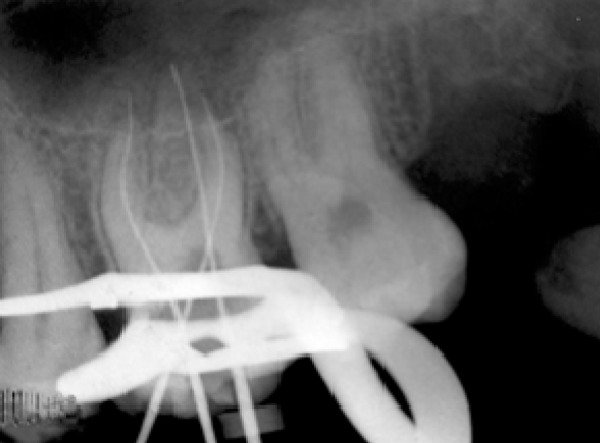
The WL radiograph of tooth #26
